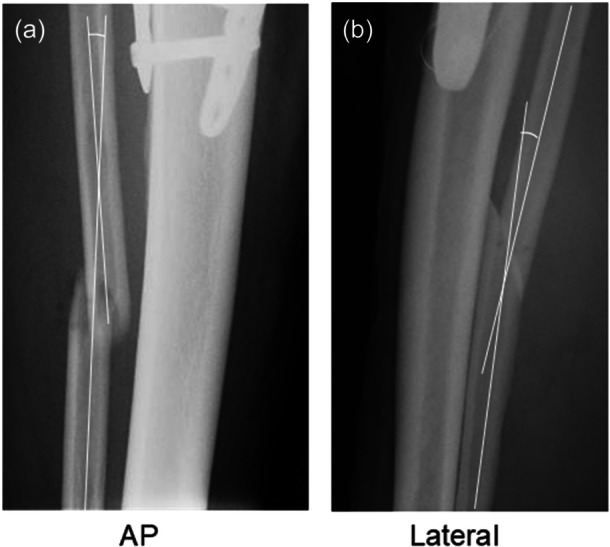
Anteroposterior (AP) and lateral radiographs of the fibula taken immediately after surgery. (a) Frontal angulation (valgus alignment). (b) Sagittal angulation (flexion alignment).
